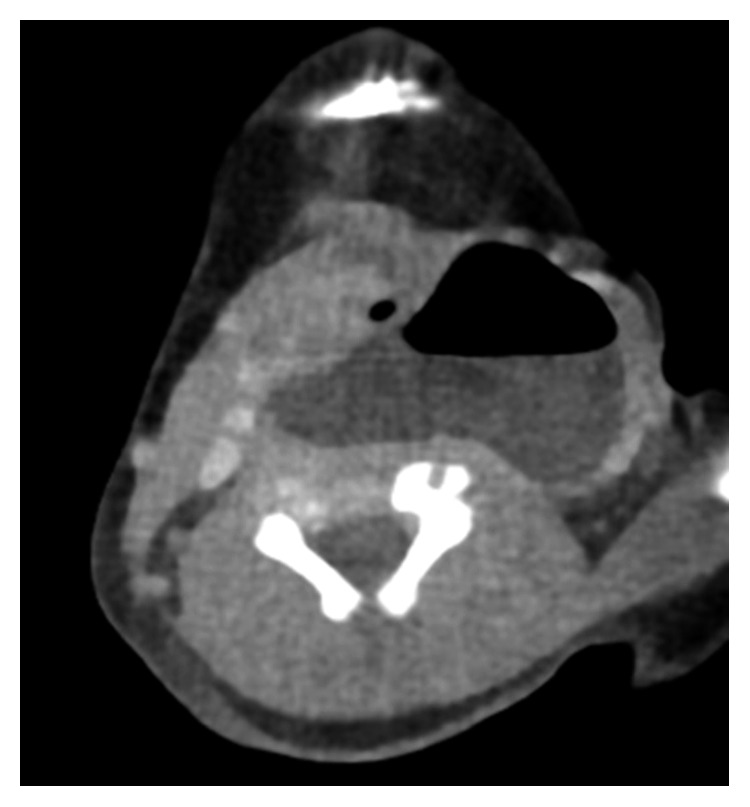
Computed tomography scan showed a cystic lesion on the left side of the neck. An air-fluid level could be detected within the cyst.
